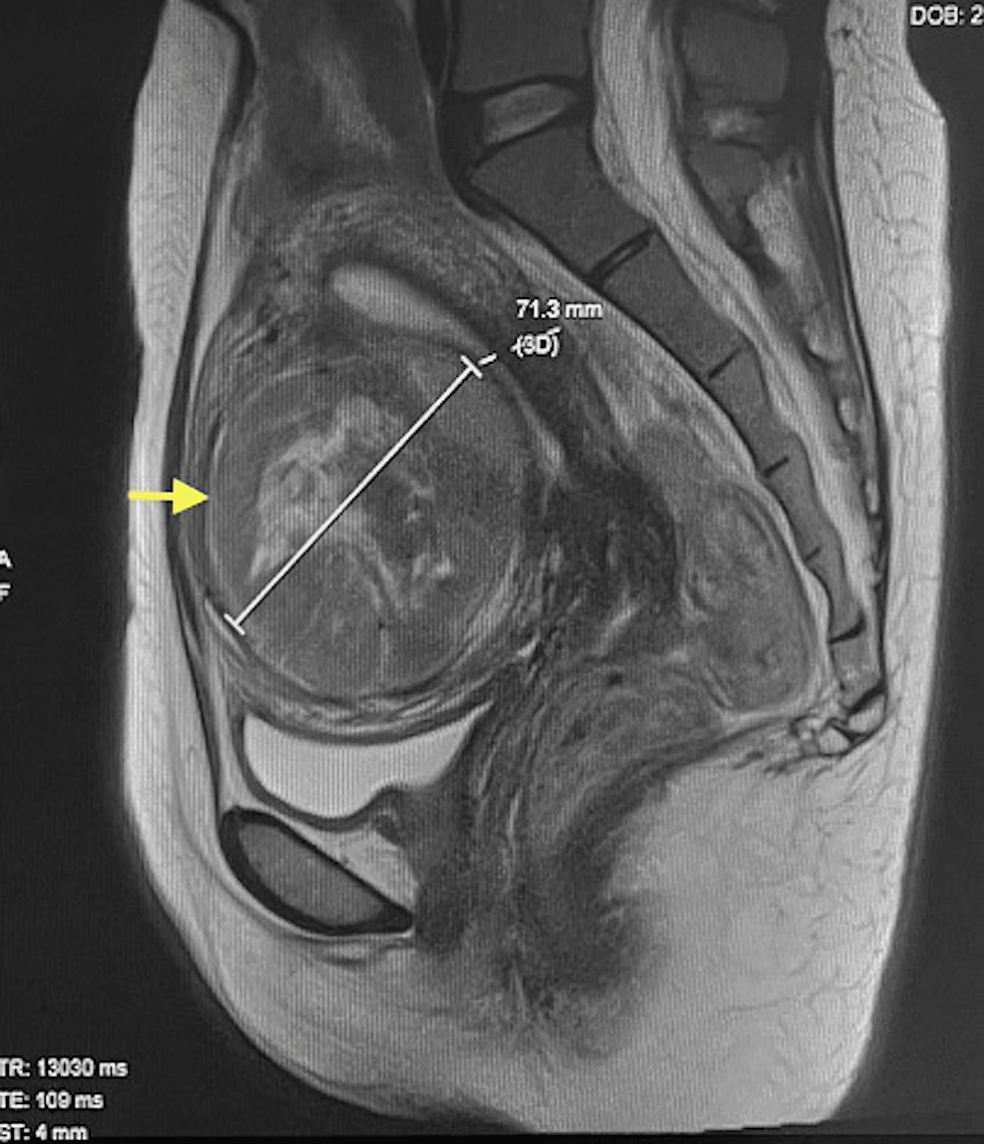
Pre-treatment MRI image Pre-treatment MRI showed an anterior FIGO Type 3 fibroid (arrow).
